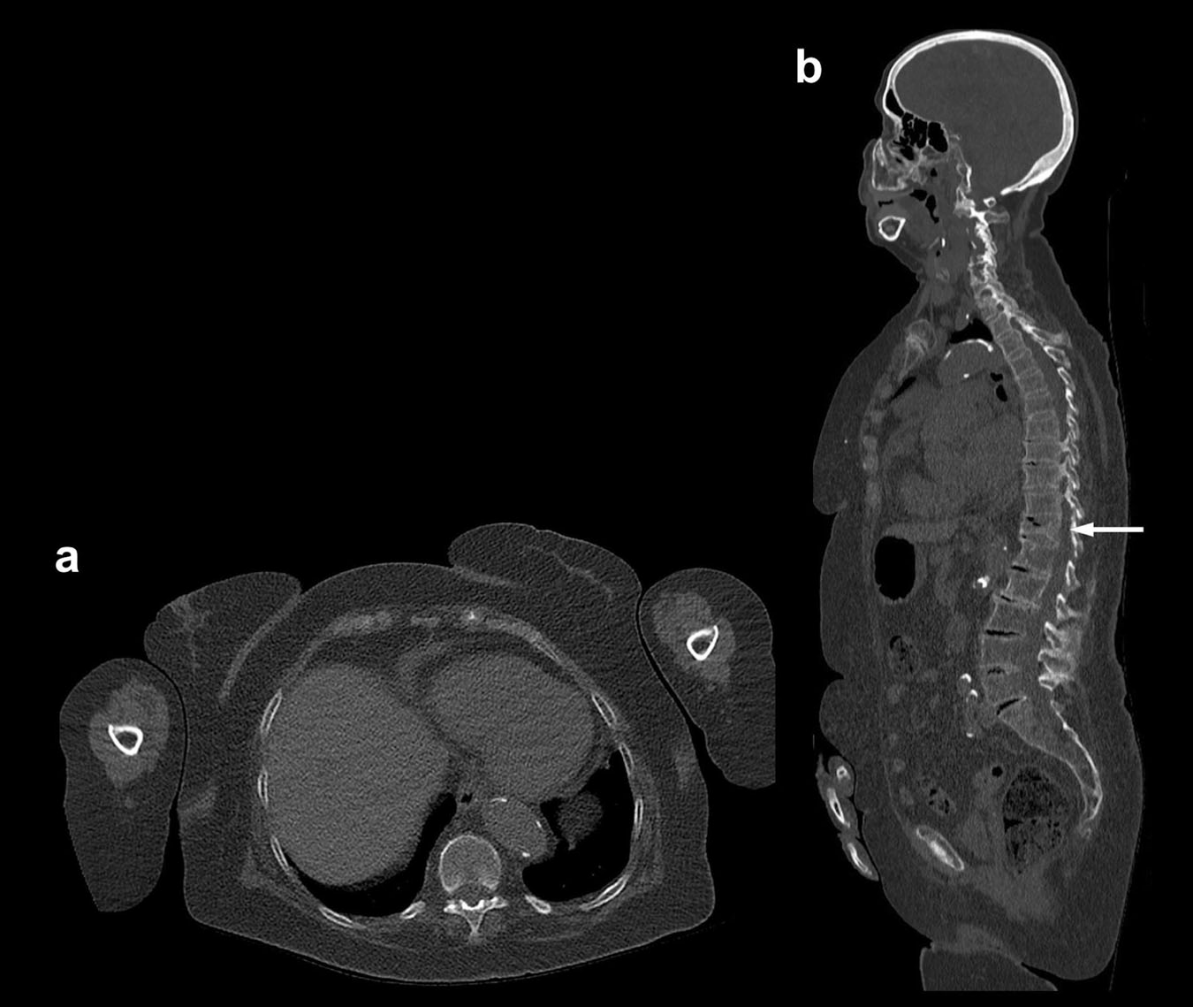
WBLDCT scan of the same patient as in Fig. 1, performed on a 128-slice CT scanner three years later with the same tube voltage and time-current product (120 kV, 60 mAs). The arms are lying more anteriorly than in Fig. 1, and an iterative reconstruction algorithm was used to reduce artifacts and image noise. a Axial slice (2/1 mm thickness/increment) at the level of the T10 vertebral body and b Sagittal MPR image. Overall image quality is significantly improved compared to the images of Fig. 1. The trabecular structure of the T10 vertebral body is readily appreciated in a and spinal anatomy is well depicted on b. Note new compressive vertebral fracture of T11 (arrow in b). *MPR* multiplanar reformation
